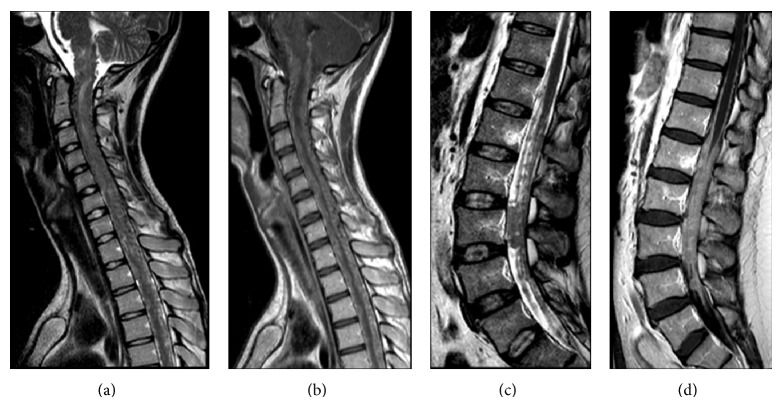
Primary leptomeningeal melanomatosis in patients 1 ((a), (b)) and 2 ((c), (d)). (a), (c): Sagittal T2-weighted images (TR/TE 3500 ms/120 ms) of cervical-dorsal spine and cauda equina show hypertrophic leptomeninges with crowded subarachnoid space and multinodular appearance of the cauda equina. (b), (d): Sagittal T1-weighted images (TR/TE 65 ms/9 ms) of cervical-dorsal spine and cauda equina show diffuse leptomeningeal enhancement and thickening.
